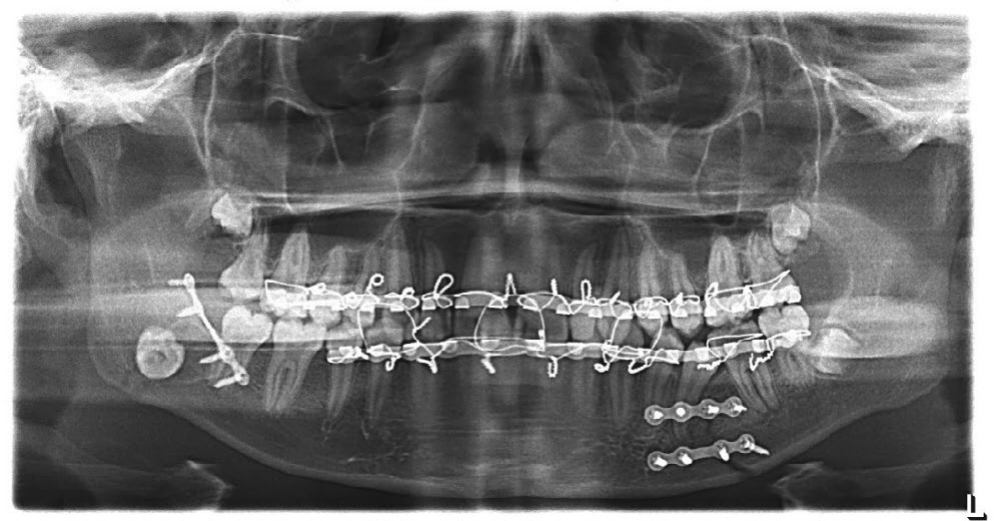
Postoperation radiographs, the displacement of the third molar bud in the panoramic view
